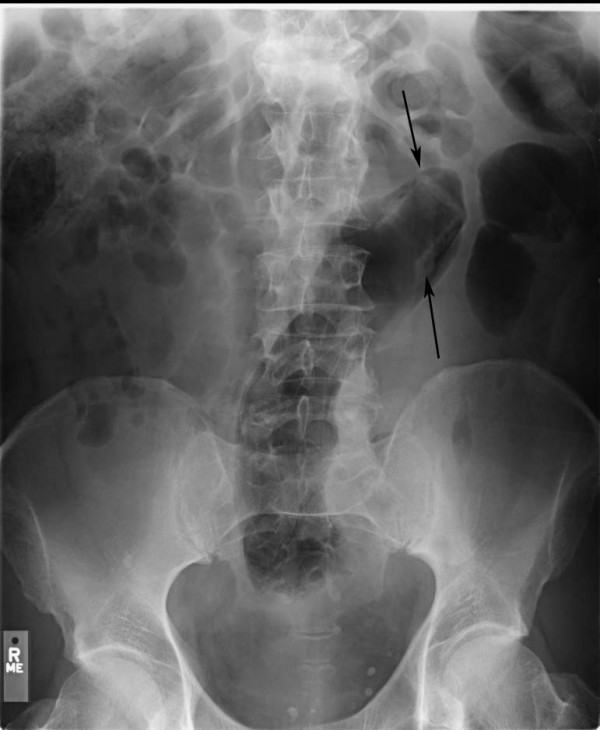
X-ray of abdomen 2 years prior showing outline of plastic soda bottle in sigmoid colon (arrows).
